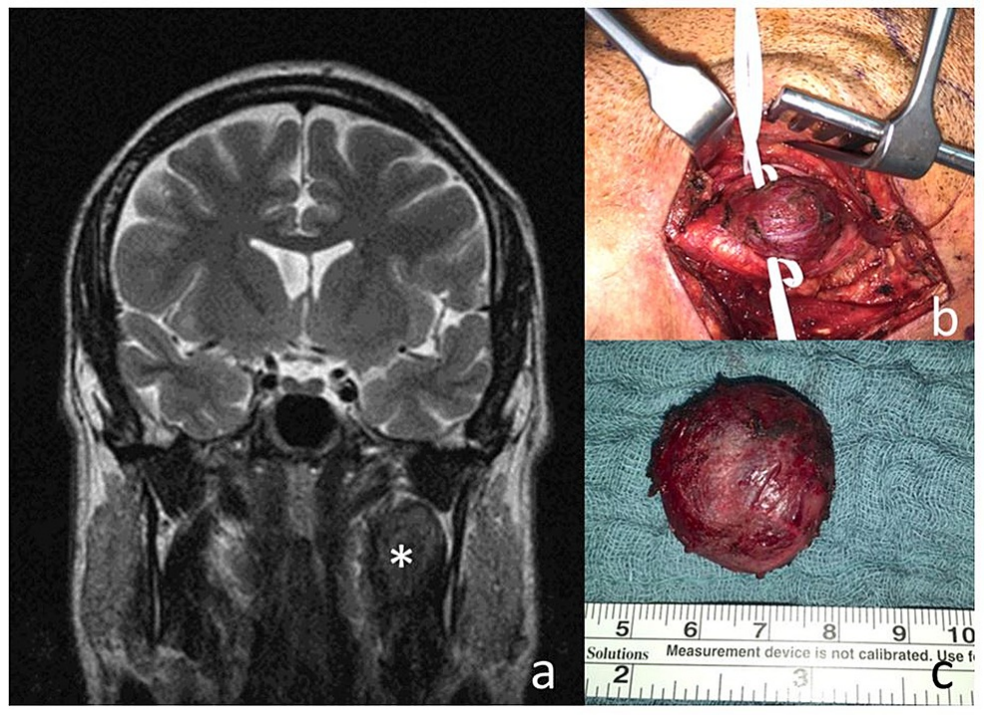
Left-sided glomus caroticum tumor in patient 5/III. (a) Coronal MRI showing the carotid body tumor (*) in the left neck. (b) Intraoperative view with vessel loops around the internal and external carotid artery; right = cranial, up = anterior. (c) In toto resected tumor.
